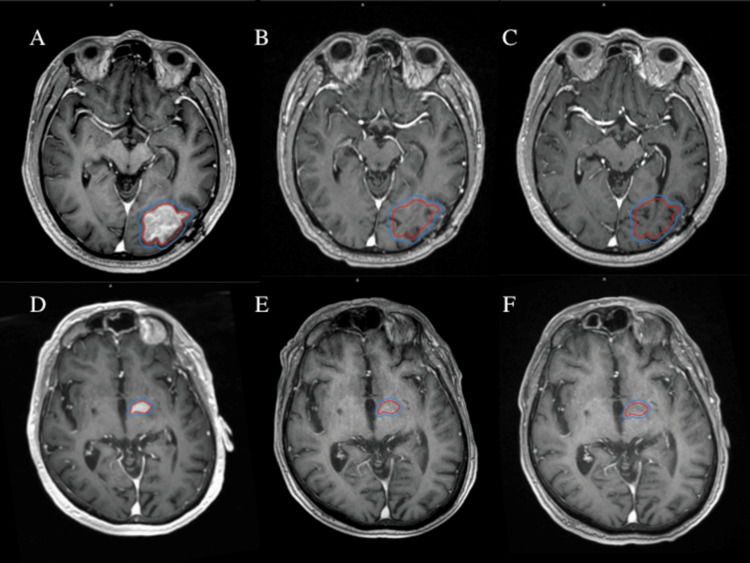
Representative axial T1 post-contrast MRI images demonstrating target delineation for linac-based radiosurgery in the treatment of PCNSL and response following SRS. GTV is contoured in red. PTV is contoured in blue. Patient one is a 46-year-old man with relapsed PCNSL following complete response to HD-MTX: (A) Planning MRI with a 3 mm expansion from GTV to PTV, prescription dose 25 Gy in five fractions. (B) Follow-up MRI images at two months and (C) four months following treatment. Patient two is a 74-year-old man with progressive PCNSL following HD-MTX, rituximab, and pomalidomide, as well as SRS to two lesions one year prior: (D) Planning MRI with 2 mm GTV to PTV expansion, prescription dose 25 Gy in five fractions, follow-up MRI images at (E) two months and (F) five months. Both patients had resolution of enhancing disease by their second follow-up MRI (C & F). PCNSL = primary central nervous system lymphoma; SRS = stereotactic radiosurgery; GTV = gross tumor volume; PTV = planning target volume; HD-MTX = high-dose methotrexate
